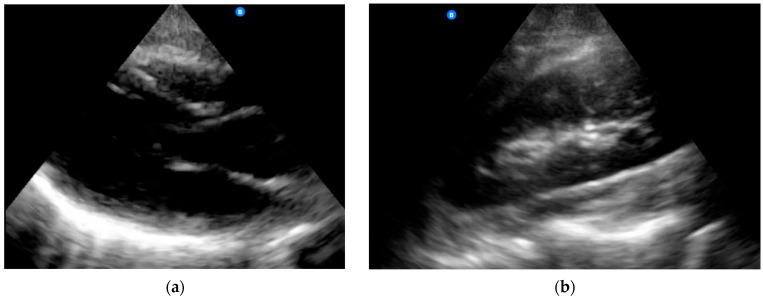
Example images obtained with the Butterfly iQ+ platform: (a) normal cardiac parasternal long axis view; (b) normal renal long axis view.
